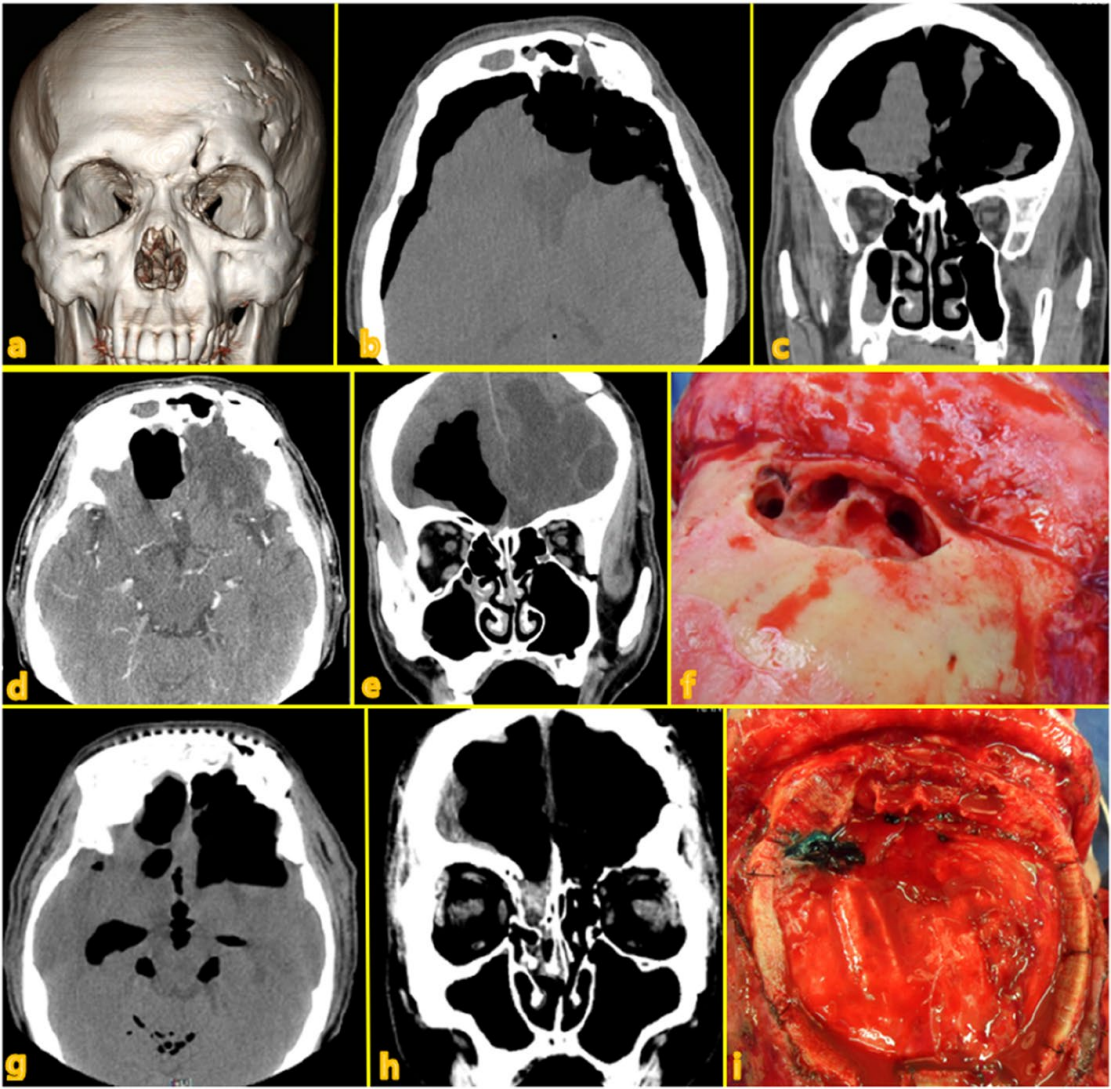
a–c CT image acquired at the first visit. Medical history of craniotomy, extensive pneumocephalus, defects in the posterior wall and floor of the frontal sinus, and channel formation between the brain and paranasal sinus. The patient refused surgery at this time and was discharged. d and e CT scan acquired during the second visit. Intracranial abscess formation and pneumocephalus can be observed. f The osteotomized anterior wall of the frontal sinus is elevated, and the eroded posterior wall can be observed. Meticulous debridement of the mucosal membrane was performed. The formed channel was identified through the eroded posterior wall. g and h CT scan image acquired during the third visit (four postoperative weeks) shows increased pneumocephalus. i Craniotomy and dural repair were performed. CT, computed tomography
